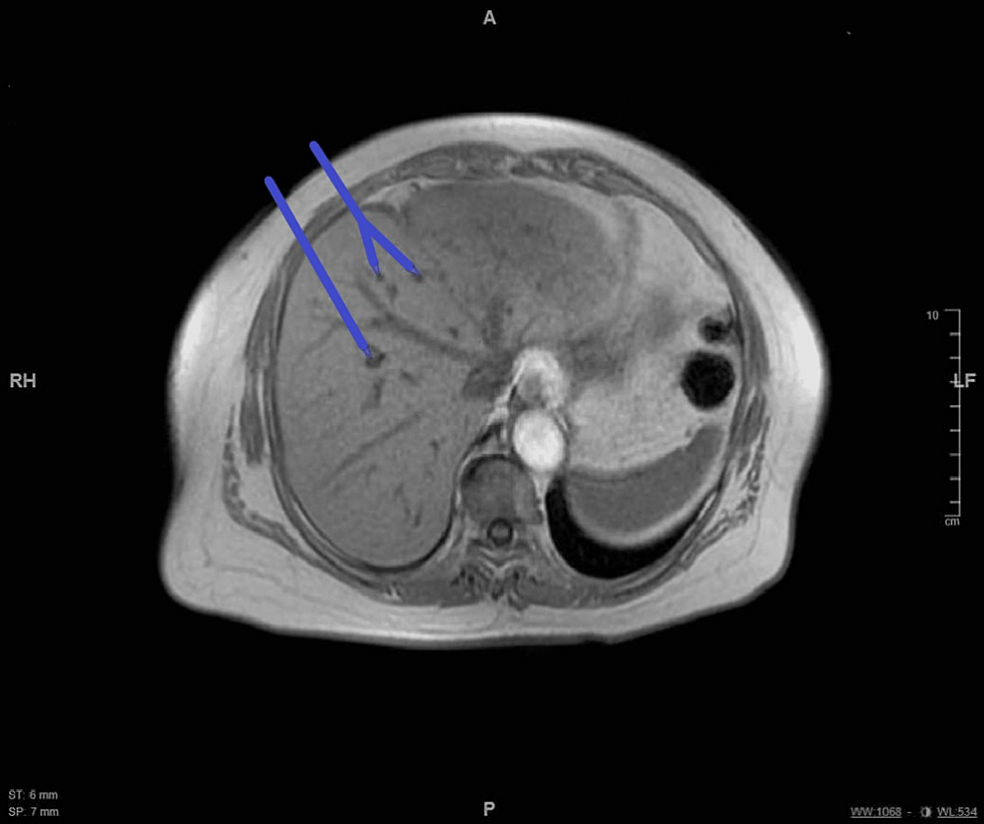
MRI: Arrows indicate the multiple hepatic cysts reported.
